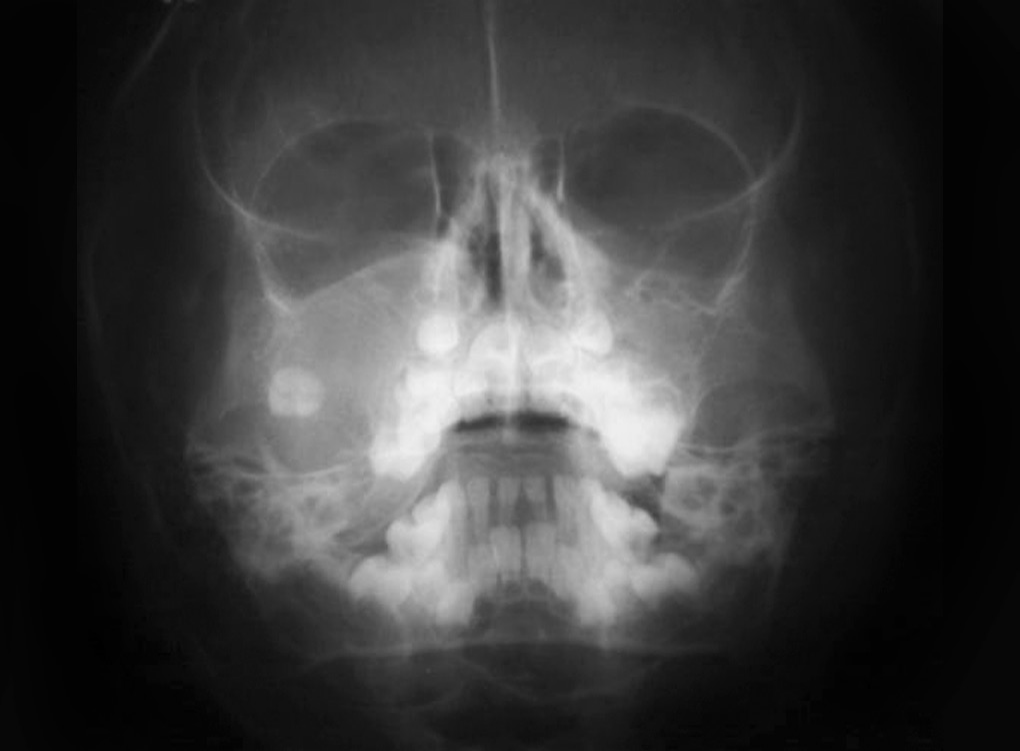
Extraoral radiograph of a lytic lesion in the right maxillary sinus, with the erosion of the lateral wall and displacement of tooth 17 distally
